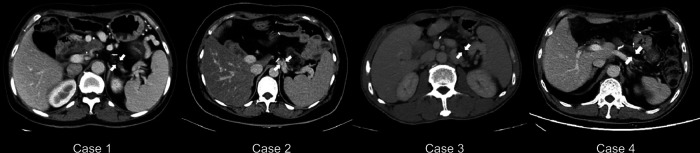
Abdominal CT scans during the follow-up studies. Abdominal CT reveals that the distal pancreas is intact in all cases (indicated by arrows). CT = computed tomography.
